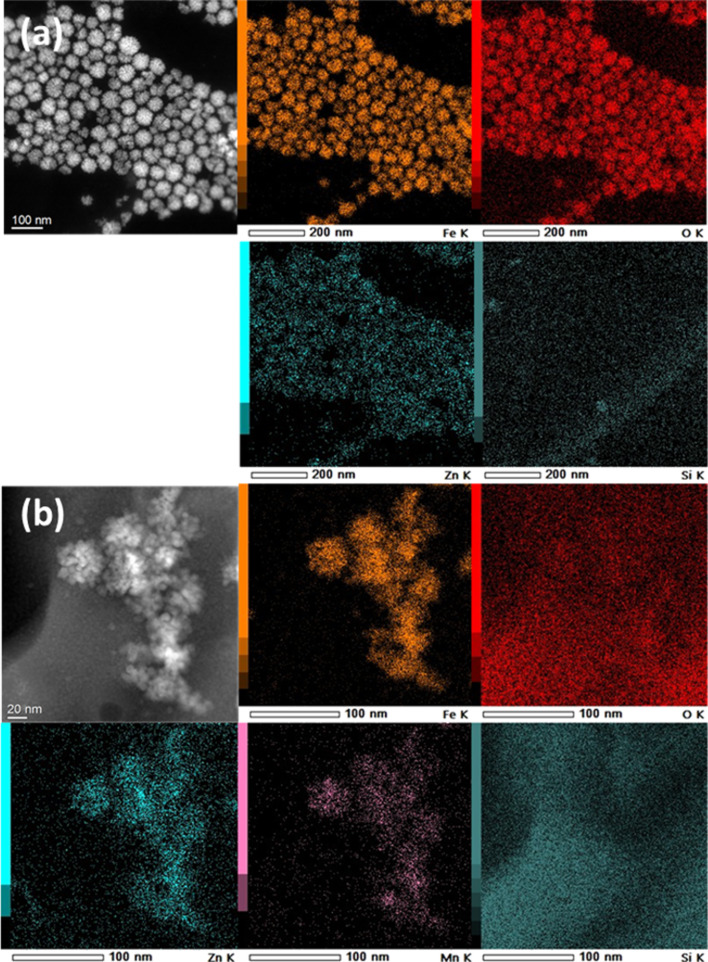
X-ray element mapping images of Zn_0.4_Fe_2.6_O_4_ NPs (45 nm) (a) and Zn_0.4_Fe_2.6_O_4_@MnFe_2_O_4_ NPs (50 nm) (b).
